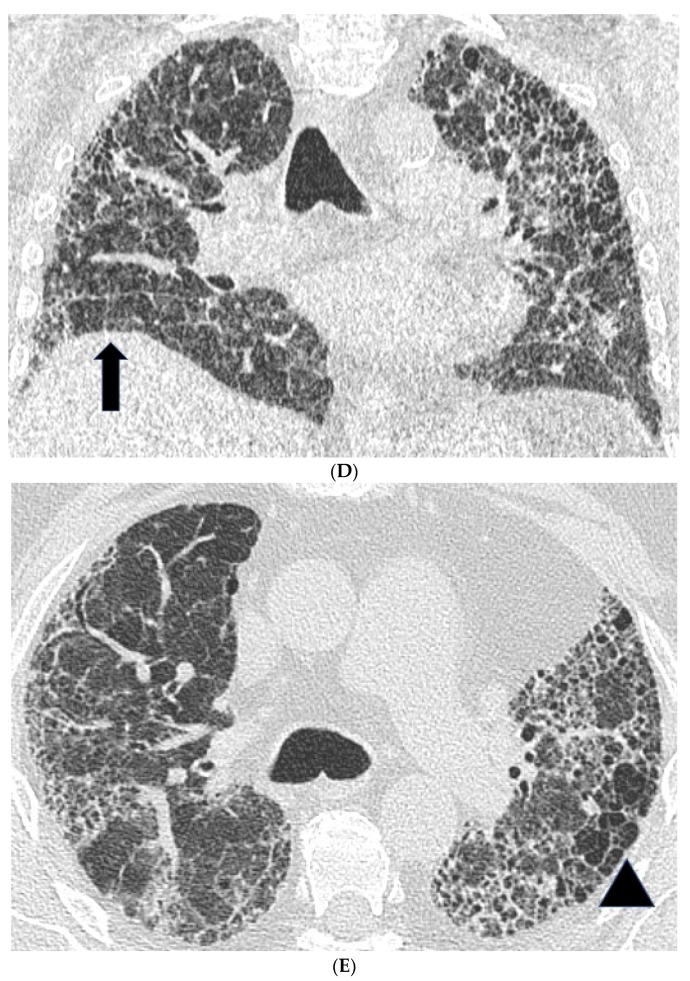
Features suggestive of chronic hypersensitivity pneumonitis in different patients. (A) Spared pulmonary lobules are visible within non-fibrotic lung bilaterally (arrows); background fibrosis is evidenced by peripheral reticulation and traction bronchiectasis (black arrowheads). (B,C) A predilection towards bronchocentricity of the fibrosis in the upper lobes is evident on axial (arrowheads) and coronal (arrows) images. (D) An upper and midzone predominance to the fibrosis, characterised by reticulation and traction bronchiectasis, is seen on a coronal CT. Incidentally, volume loss in the right lung is noticeable by slight tenting and elevation of the right hemidiaphragm (arrow). (E) A UIP pattern with honeycomb cysts is visible in the left midzone of the lung (arrowhead) in a 74-year-old male ex-smoker. A surgical biopsy performed a few years before this CT had demonstrated findings compatible with fibrotic hypersensitivity pneumonitis.
